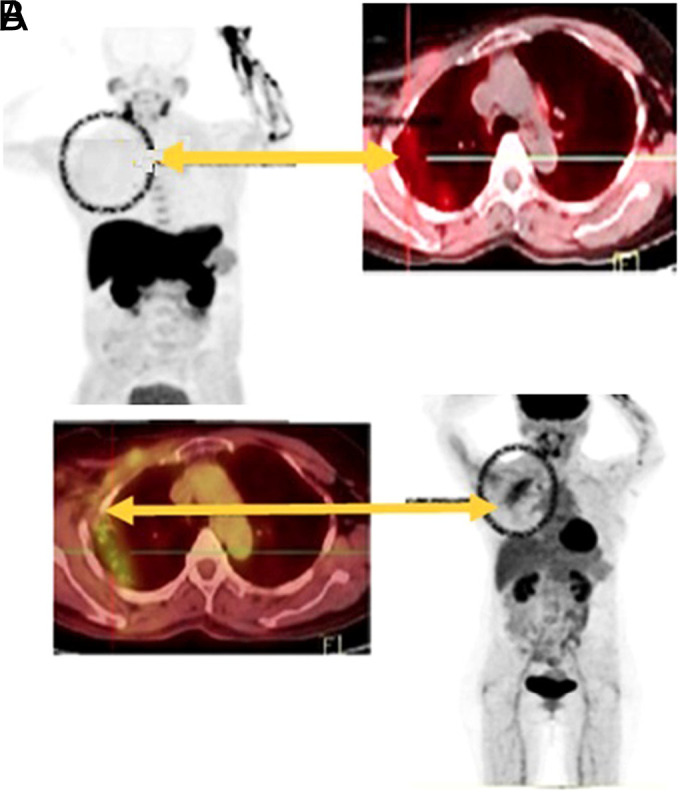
A 60-year-old female with human epidermal growth factor receptor 2 +ve right breast tumor (T3N0M1) post right mastectomy and radiotherapy. Coronal (A) MIP positron emission tomography (PET) (A): [18]F-fluorocholine (FCH) revealed no evidence of altered phospholipid metabolism denoting radiation pneumonitis as shown on the [18]-F-fluorodeoxyglucose (FDG) image axial (B) fused PET-computed tomography. The added value of the [18]F-FCH is that it dismisses false-positive changes of a metastatic tumor as noted on the correlated FDG-avid radiation pneumonitis.
